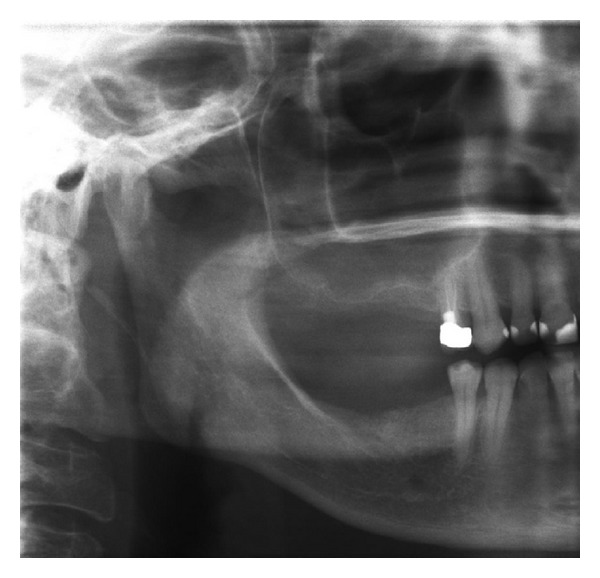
Case 2: presurgical condition in panoramic X-ray.
